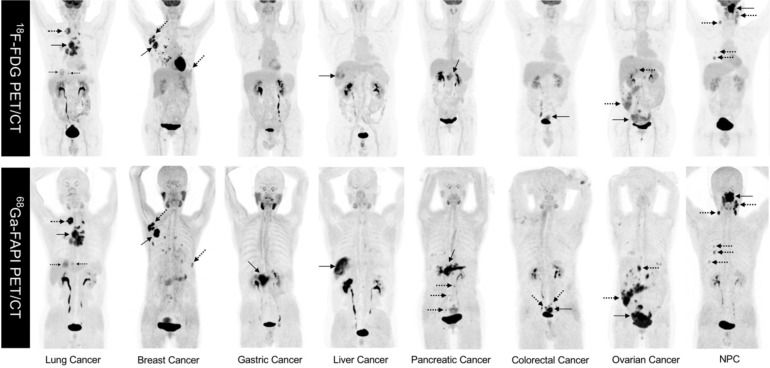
Representative comparison of 8 patients with different tumor entities undergoing both ^18^F-FDG PET and ^68^Ga-FAPI-04 PET imaging within less than 1 week. Solid arrows indicate primary tumors, while the dotted arrows indicate metastasis lesions. NPC: nasopharyngeal carcinoma.
